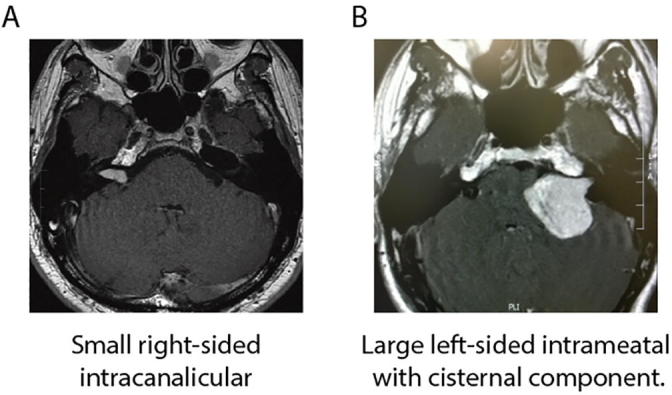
MRI scan of vestibular schwannoma. (A) A patient with a small right-sided intracanalicular tumour. (B) A patient with a large left-sided intrameatal tumour with a cisternal component.
